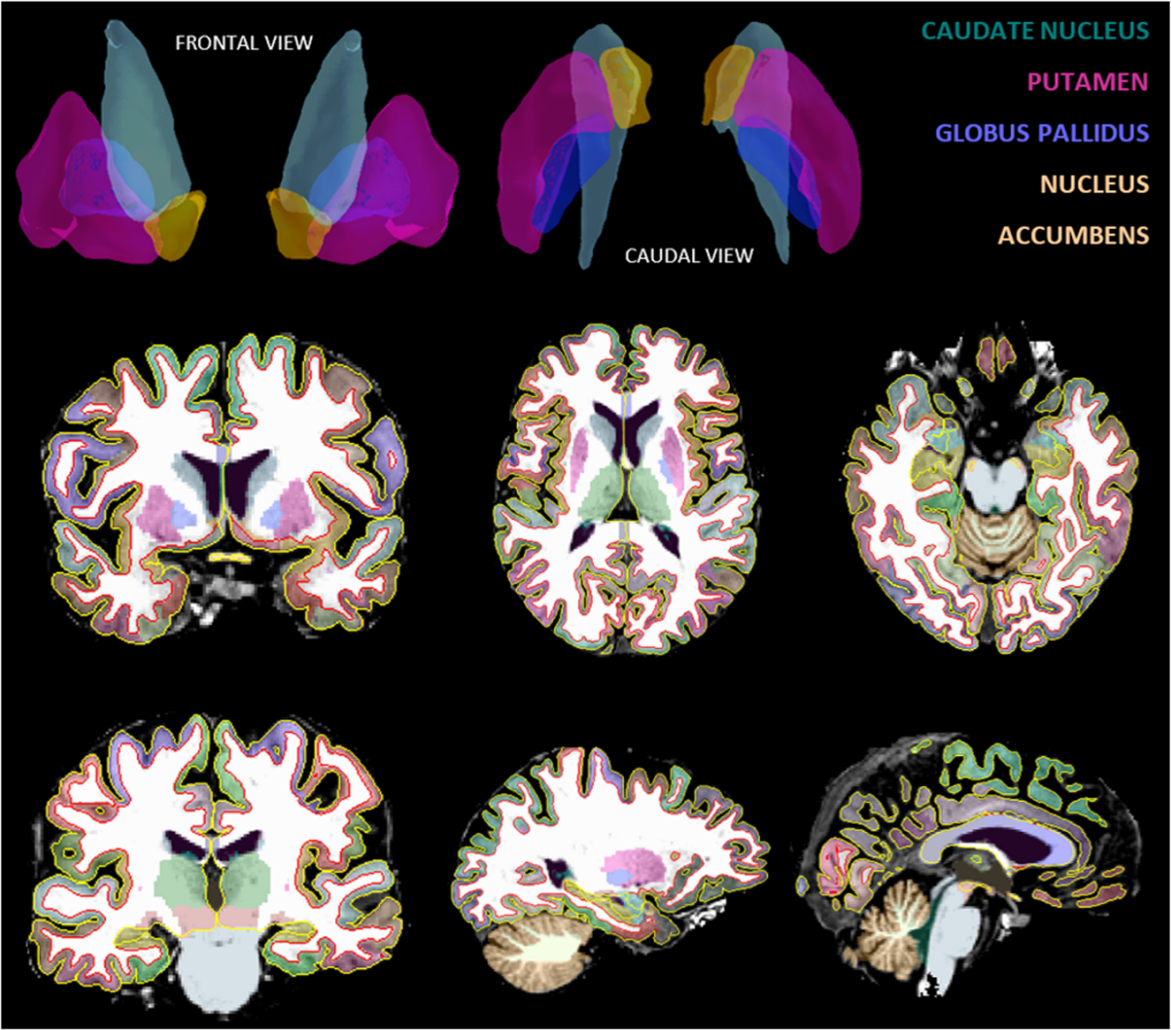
Volumetric 3D segmentation of high-resolution 3D T1-weighted MR images using Freesurfer 6.0.0 (Fisher et al. 2012). Caudate nucleus, putamen, globus pallidus, and nucleus accumbens volumes were extracted from whole-brain segmentation images. Participants were scanned at three time points: prior to ECT (baseline, *t*_0_), one week after (*t*_1_), and six months after ECT completion (*t*_2_). The volumetric data were normalized to account for differences in total brain volume using a standard approach (Jack et al.^48^).
